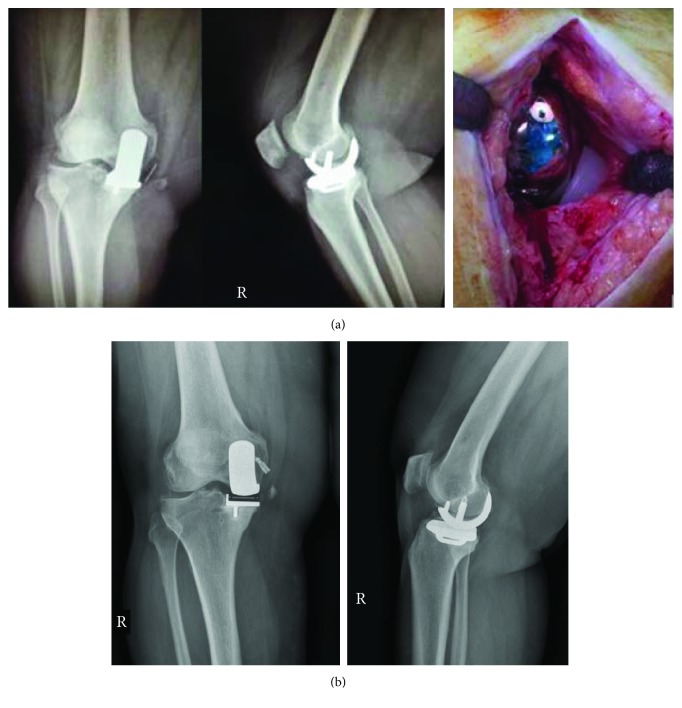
(a) X-ray and preoperative images of insert dislocation. (b) Postoperative 2nd year AP lateral standing.
